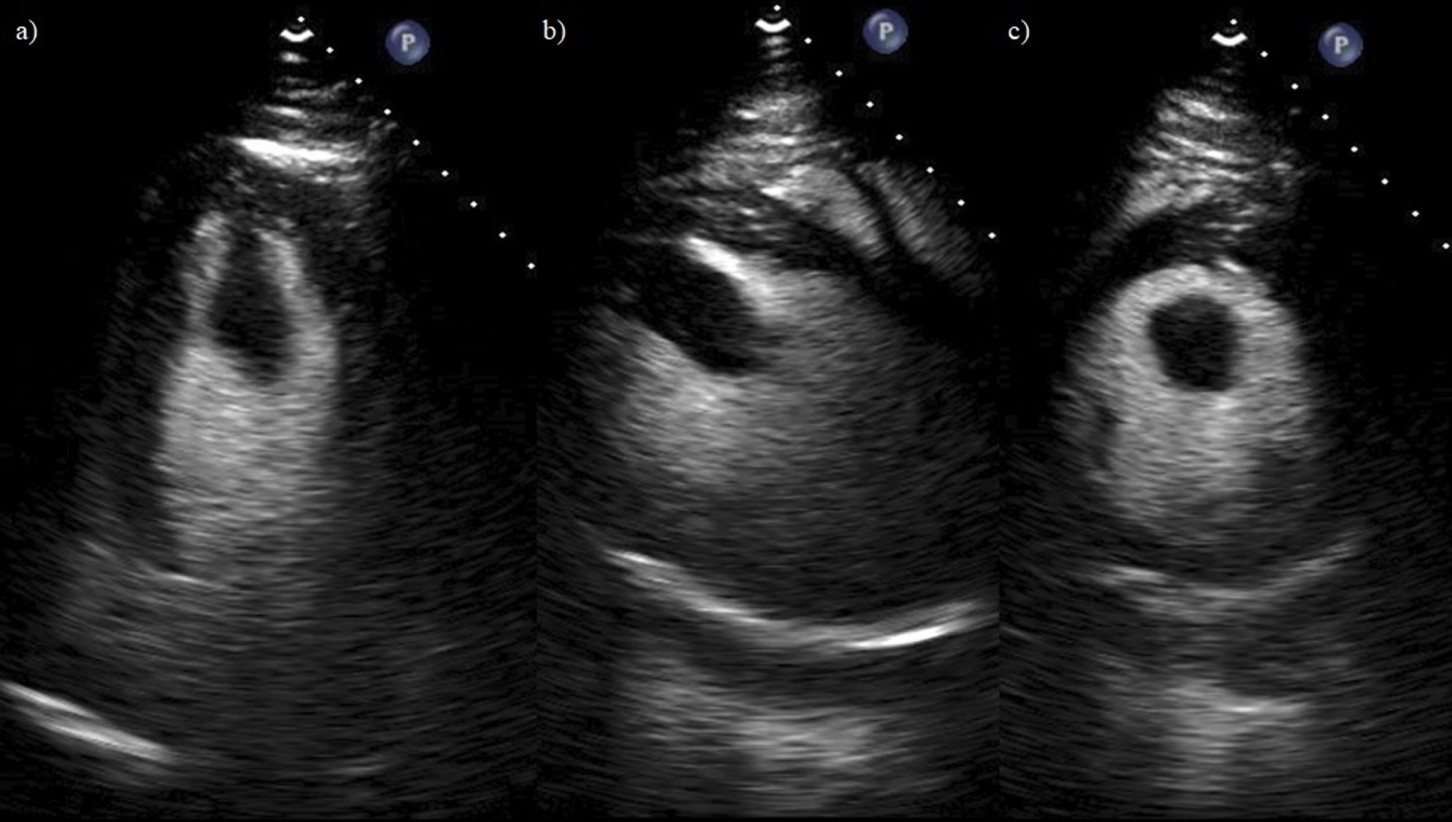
Transthoracic echocardiogram with perflutren lipid microsphere contrast. (A) Perflutren lipid microsphere contrast-enhanced apical four-chamber view of the hyperechoic, pedunculated, and mobile apical mass. (B) Perflutren lipid microsphere contrast-enhanced left parasternal long-axis view of LV apical mass. (C) Perflutren lipid microsphere contrast-enhanced left parasternal short-axis view of LV apical mass.
